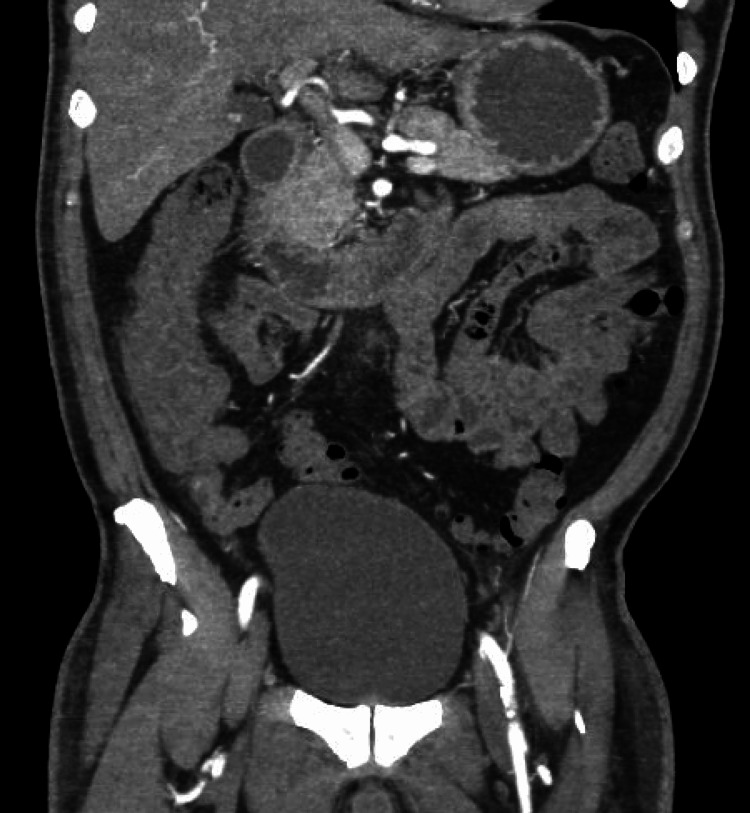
Coronal view of CT Abdomen & Pelvis showing colitis of ascending colon
